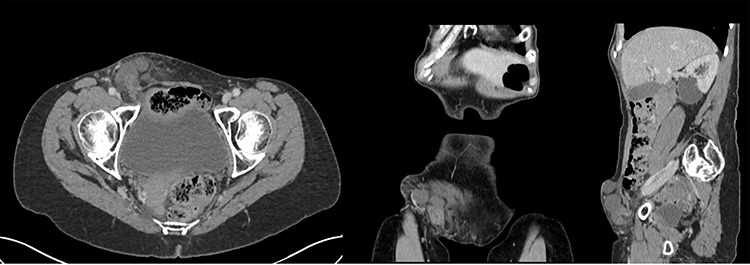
CT scan images of a 68-year-old woman who presented with acutely inflamed appendix within a right inguinal hernia sac (Amyand’s hernia) during COVID-10 pandemic.
